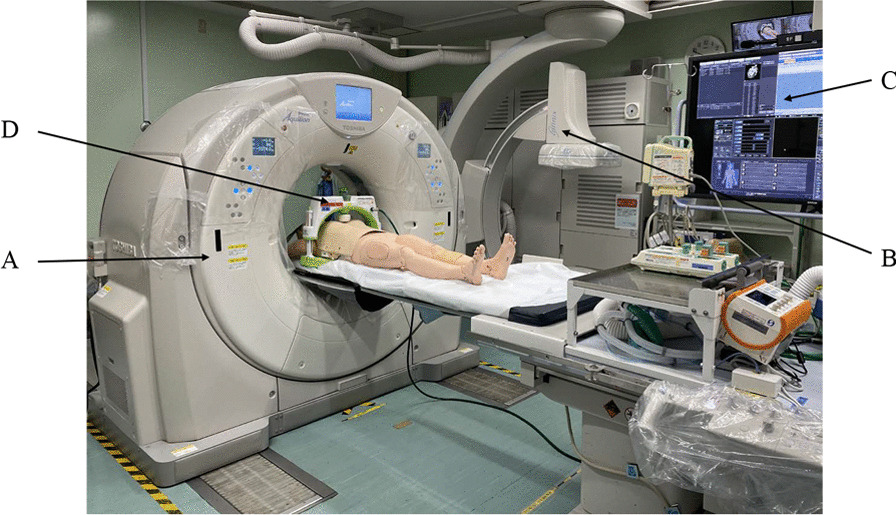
This photograph shows our hybrid ER and the scene while performing CT under mechanical CPR. A Sliding gantry computed tomography scanner, B movable C-arm, C monitoring screen, D automatic chest compression device. *ER* Emergency room, *CT* Computed tomography, *CPR* Cardiopulmonary resuscitation
